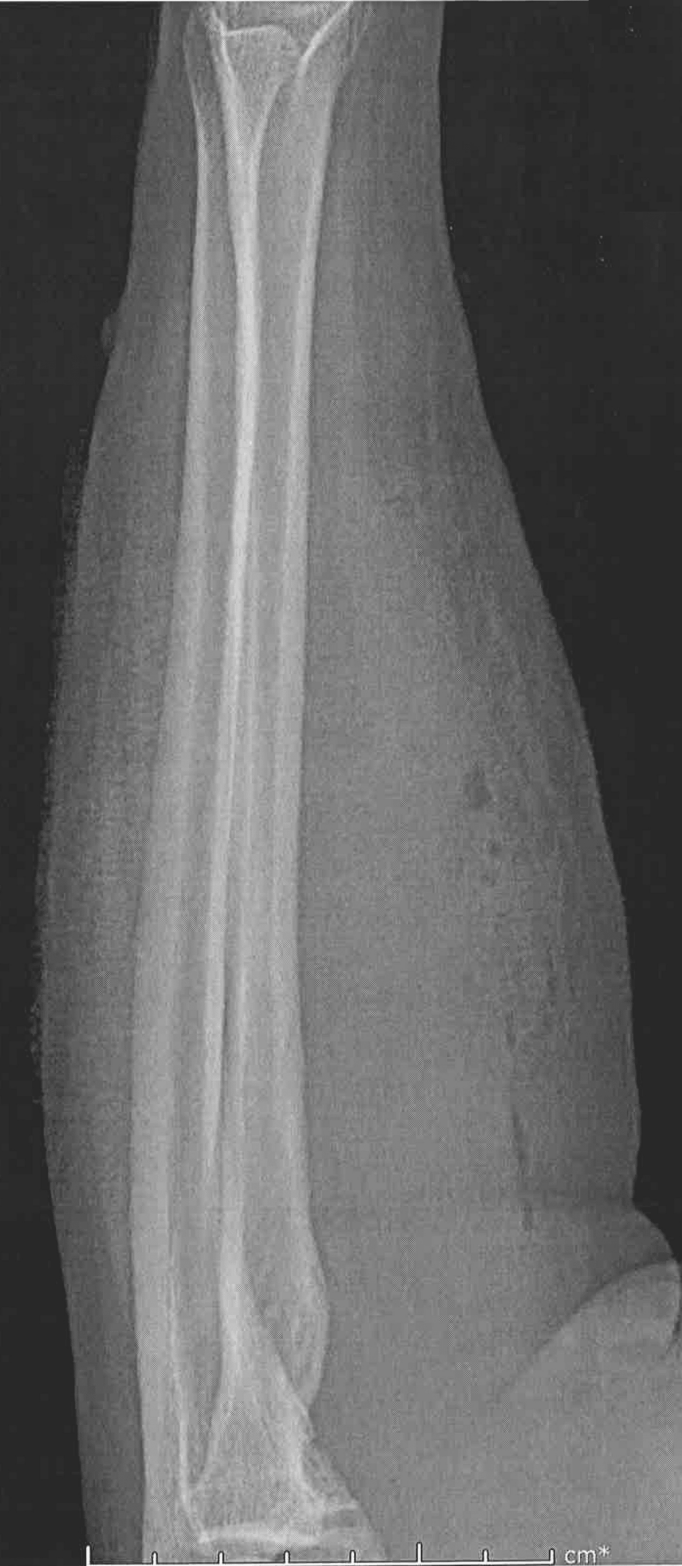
X-ray of left forearm displaying crepitus lateral view
